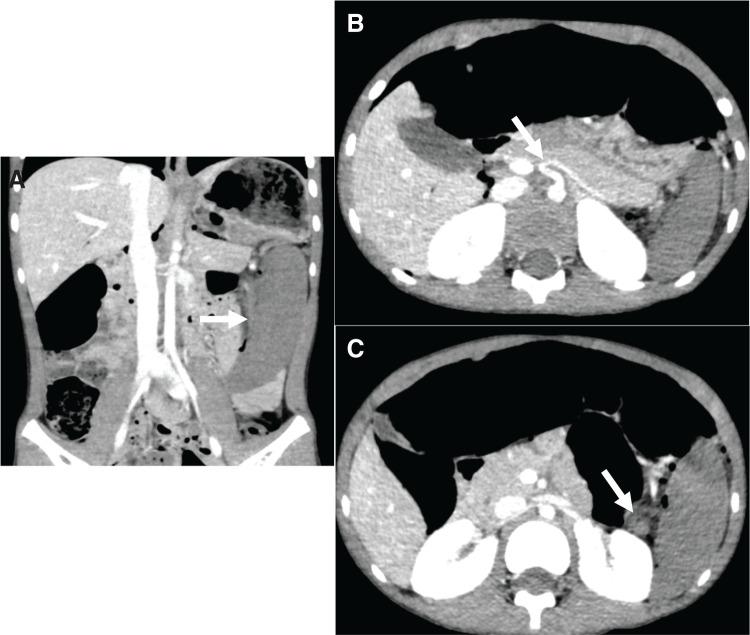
Abdominal CT scans (A) The coronal image reveals nonenhancement of the spleen (white arrow). (B) The axial image reveals the blood flow from the celiac artery to the main splenic artery (white arrow). (C) The axial image reveals the twisting of the blood vessels and disruption of blood flow at the splenic hilum (white arrow).
